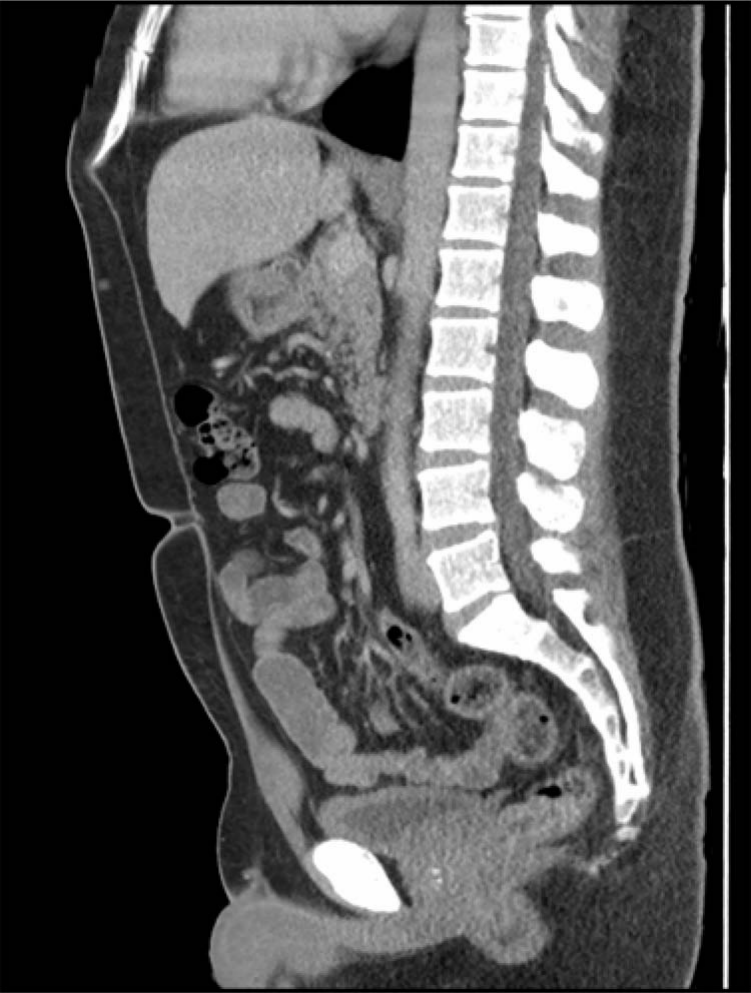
Sagittal CT image. No obvious abnormalities at the dome of the bladder.
